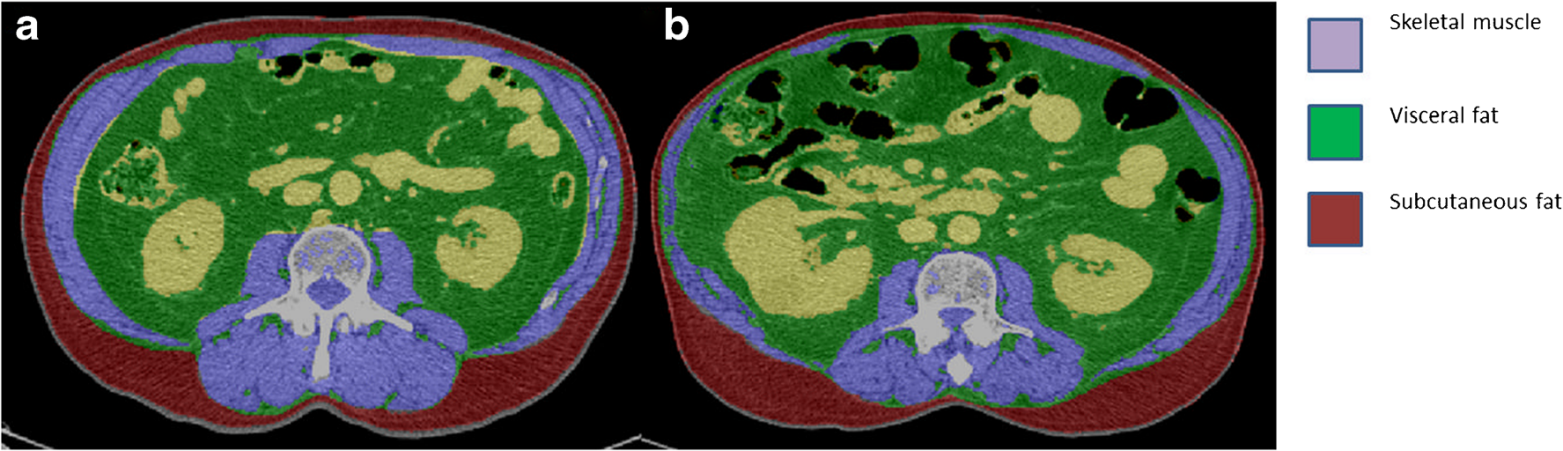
Axial CT images at the level of L3 vertebra demonstrating progressive sarcopenia in a patient with oesophageal cancer before (a) and after (b) neoadjuvant chemotherapy. There is a loss of abdominal muscle mass with an increase in visceral fat
